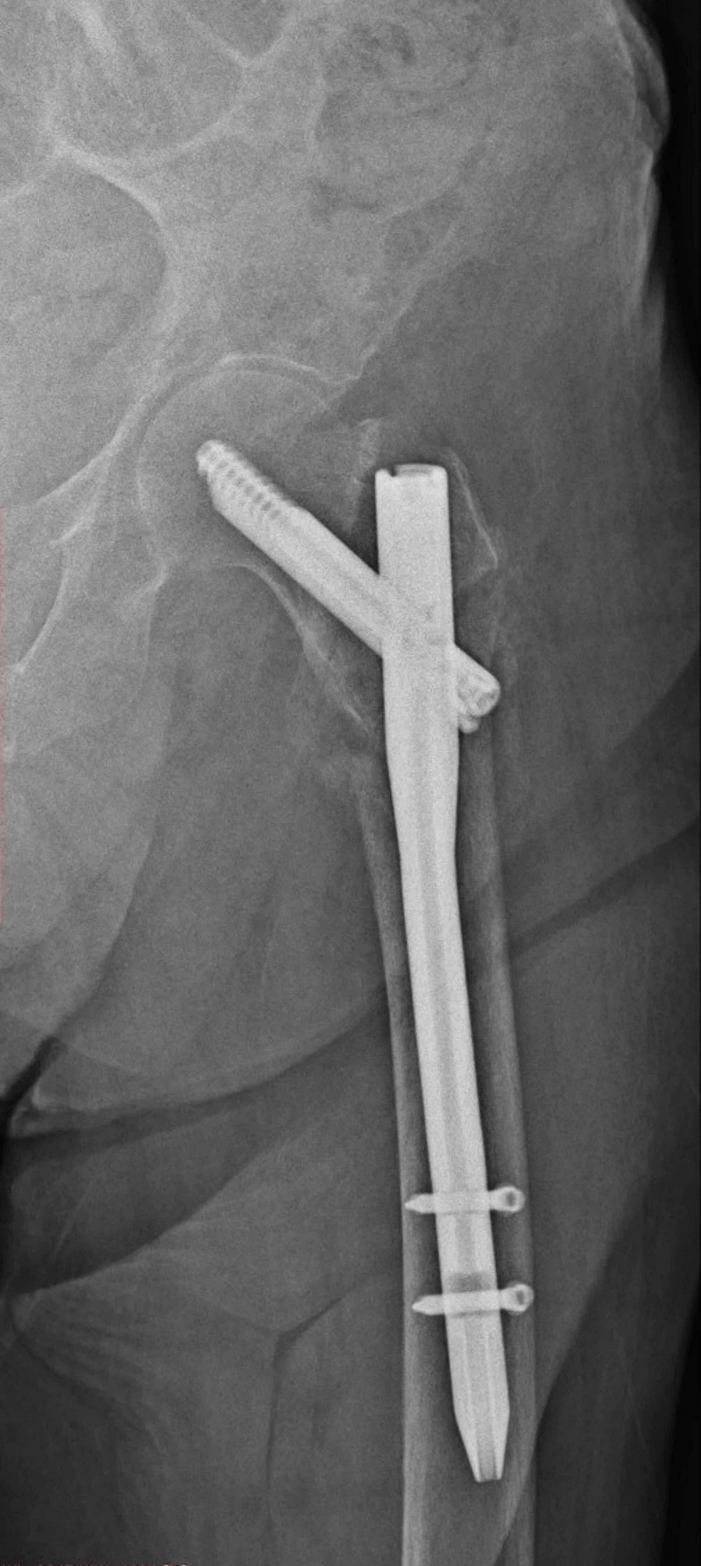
Anterior-posterior hip radiography at the third postoperative month of the patient
